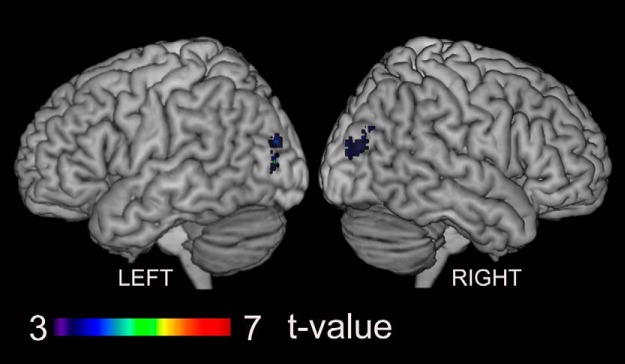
Regions exhibiting greater interbrain synchronization during the LIVE condition than the REPLAY condition. These areas are superimposed on a surface-rendered high-resolution anatomic MRI normalized to the MNI template viewed from the left and right.
